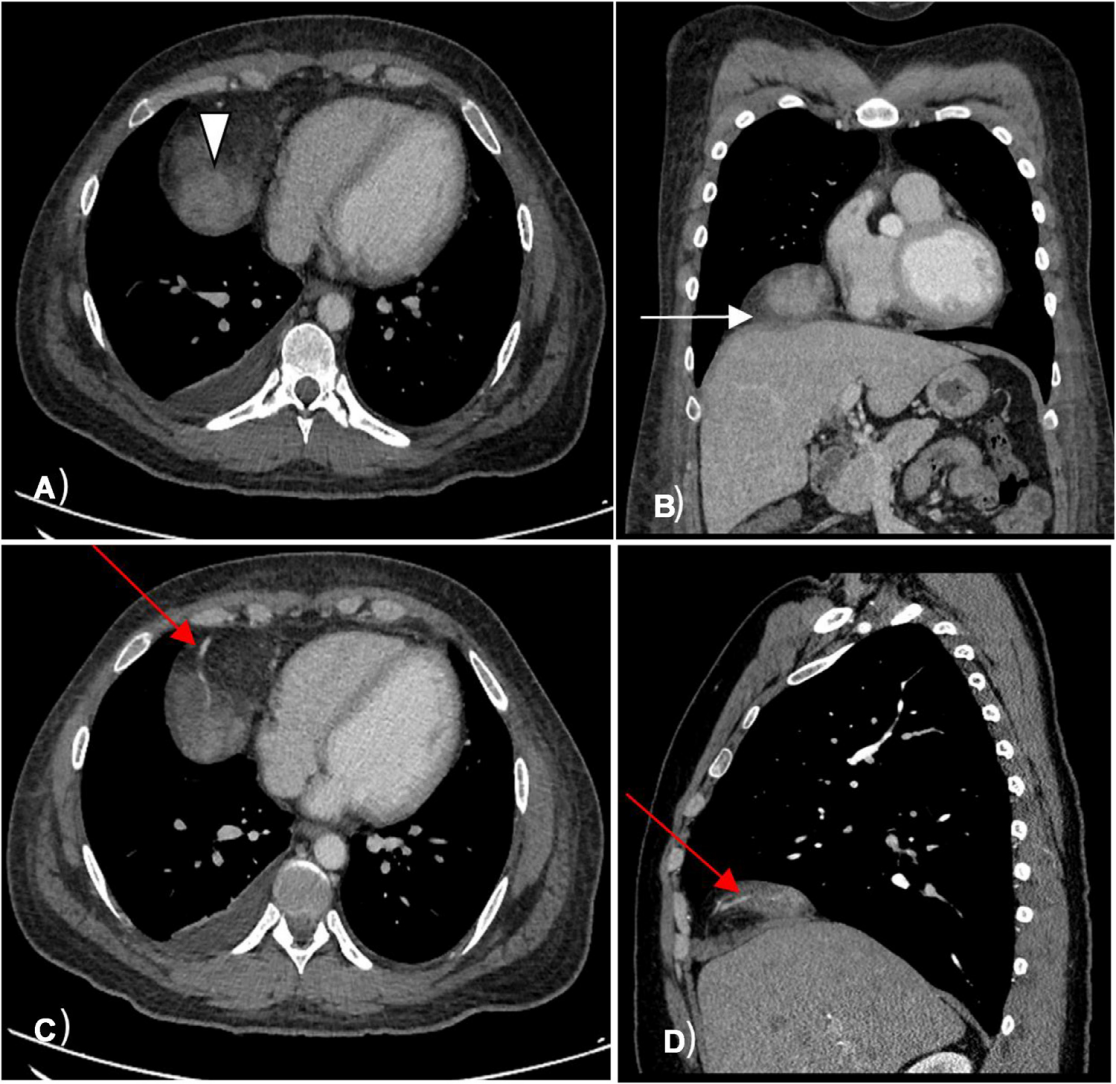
Axial CT image of the lower chest (A) demonstrates soft tissue mass in the right cardiophrenic recess (white arrowhead); Coronal CT image of the chest (B) demonstrates infiltration of the right hemidiaphragm by the mass (white arrow); Axial CT image of the lower chest (C) demonstrates a feeding vessel into mass (red arrow) that is seen as well in the sagital CT image of the chest (D).
